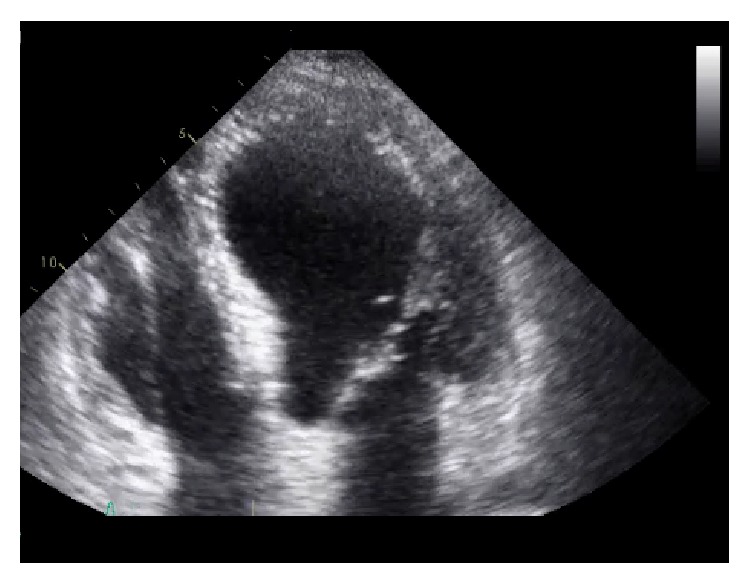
Echocardiogram with severely decreased EF of 13% and possible stress cardiomyopathy. PAP systolic 40 mmHg. Apical ballooning involving all left ventricular (LV) wall.
